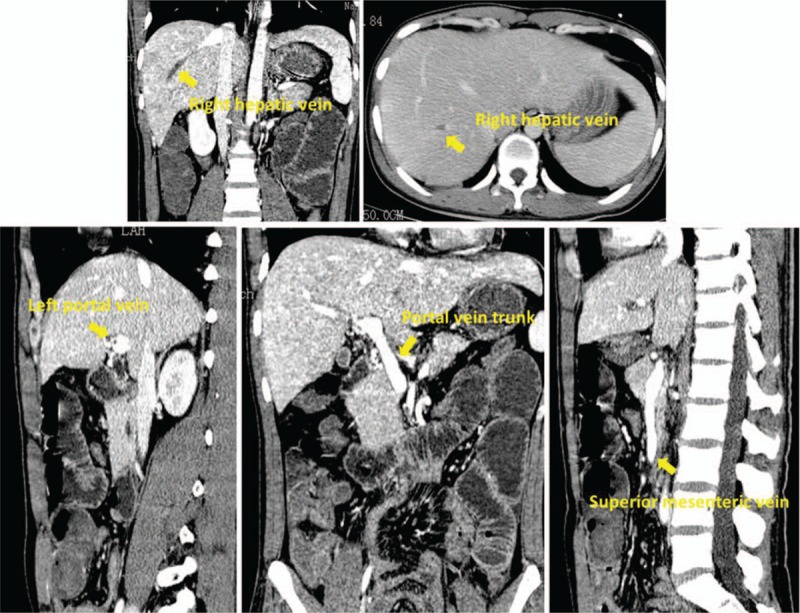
Whole abdomen enhanced computed tomography scan on July 31, 2017. Apart from the lack of significant improvement in the right hepatic vein thrombosis and small emboli in the left branch of the portal vein, the remaining veins were basically unobstructed. However, the small intestine showed expansion and effusion. A part of the small intestine was severely expanded with decreased perfusion, and intestinal necrosis could not be ruled out.
